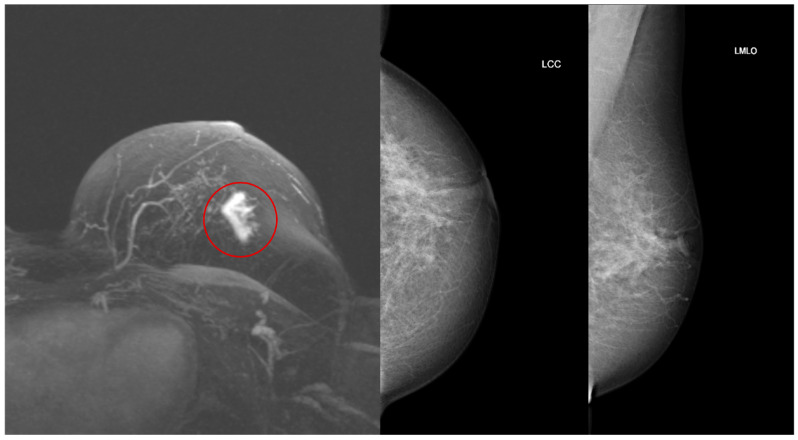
Maximum intensity projection image of breast MRI (left), and craniocaudal (LCC) and mediolateral oblique (LMLO) mammograms (middle and right) in a patient with a new malignancy in the left breast. The mass is visible on MRI (red circle) but not detected on mammography.
